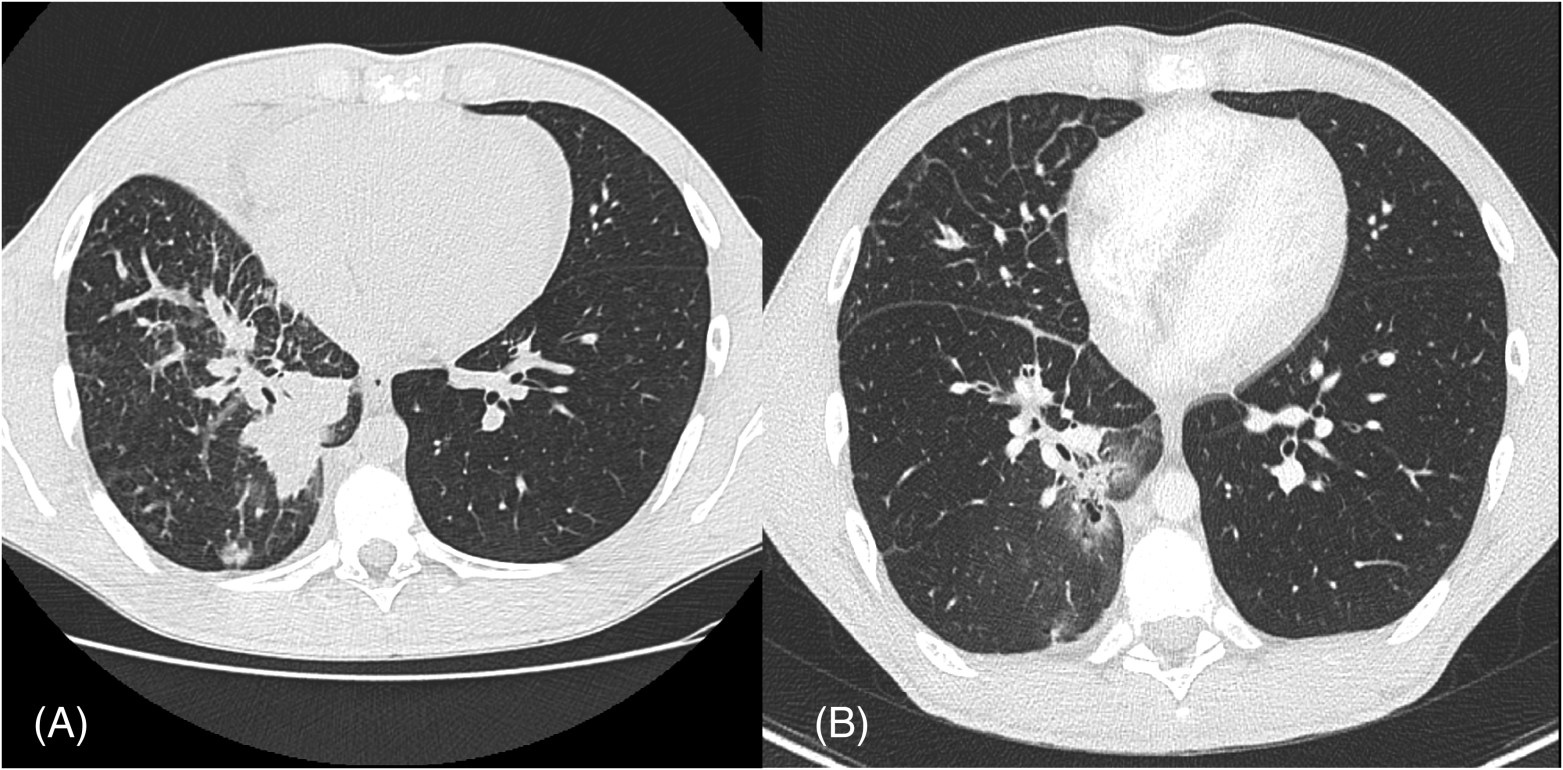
CT scan of the lungs. Adenocarcinoma with consolidation, ground‐glass opacity, centrilobular nodules, interlobular septal thickenin, before treatment (A) and after 2 months of Crizotinib (B)
